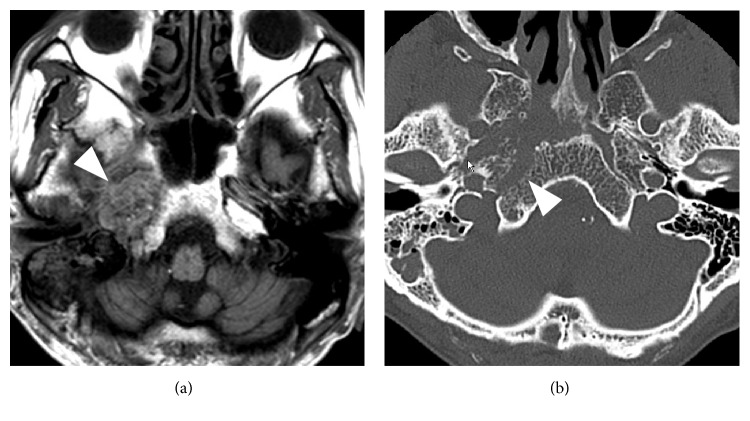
(a) T1-weighted MRI displayed a low-signal intensity lesion at the right skull base (arrowhead), replacing the high-signal intensity area of fatty tissues in the bone marrow and extending to the soft tissues inferior to the skull base. (b) CT showed erosion of the cortical bone in the right central skull base (arrowhead). Opacification of the right mastoid air cells was also noted.
